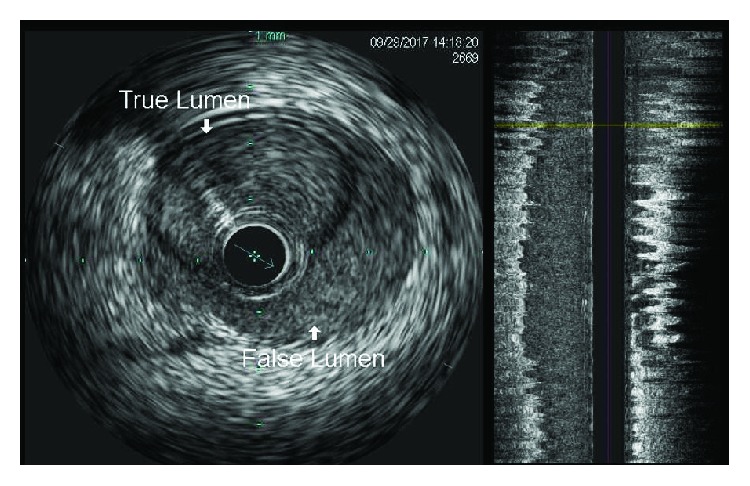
Intravascular ultrasound (IVUS) demonstrating the true and false lumens of coronary artery dissection.
